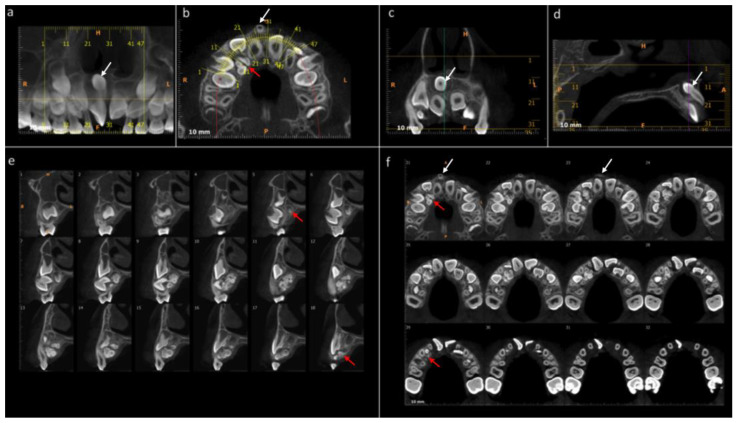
Cone-beam computed tomography (CBCT) of the maxilla. 2016, 6 years old, prior to first surgery. (a) The panoramic reconstruction shows the presence of a mesiodens supernumerary tooth between 11 and 21. (b) Axial section. Location of mesiodens vestibular to teeth 11 and 21. Presence of multiple denticles compatible with odontoma, in intraosseous evolution, distal to tooth 11, and palatal to teeth 12 and 13. (c) Coronal section. Presence of mesiodens in relation to teeth 11 and 21, surrounded by a radiolucent demarcation area. (d) Sagittal section. Presence of supernumerary, crown in apical position and root in coronal area. Presence of pericoronary sac. (e) Sagittal section. Presence of multiple radiopaque masses with irregular margins compatible with odontoma (Sections 5–18) in intraosseous evolution palatal to tooth 13. (f) Axial section. Presence of mesiodens (Sections 21–23) in vestibular relation to teeth 11 and 21. Presence of radiopaque masses compatible with odontoma (Sections 21–29) distal to tooth 11 and palatal to teeth 12 and 13. White arrow = mesiodens. Red arrow = odontoma.
